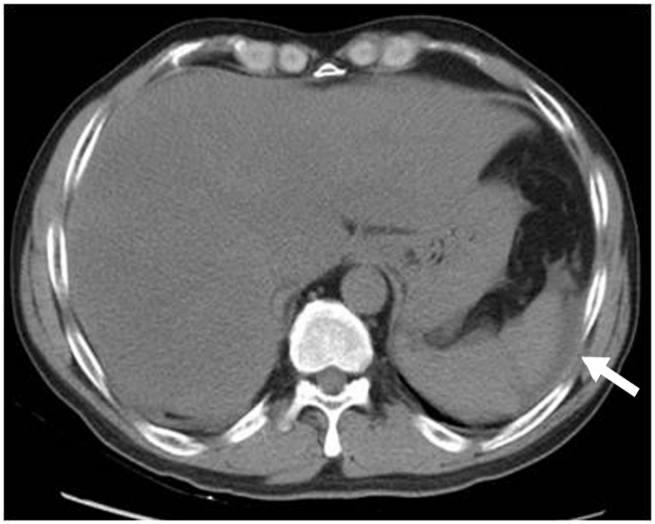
Non-contrast computed tomography of the abdomen on admission showed generally decreased liver parenchymal attenuation and mild ascites (arrow), compatible with acute hepatitis.
